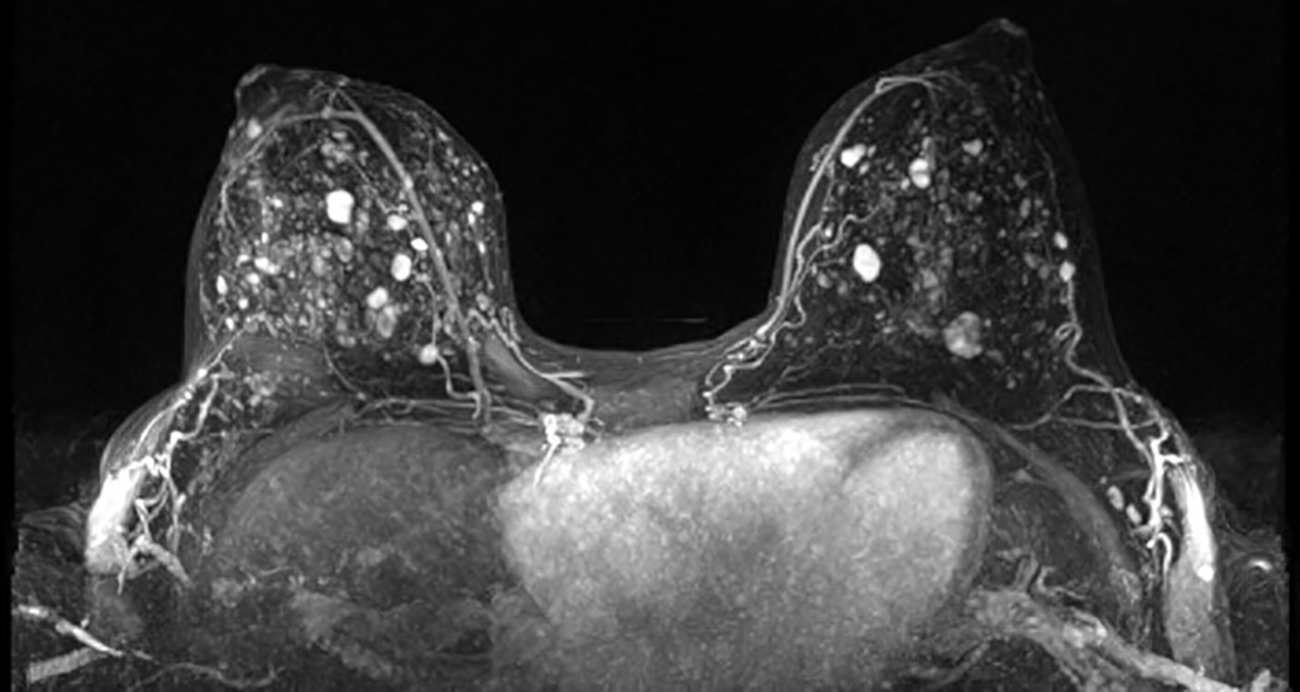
3D-MIP breast MRI showing bilateral enhancing lesions.
